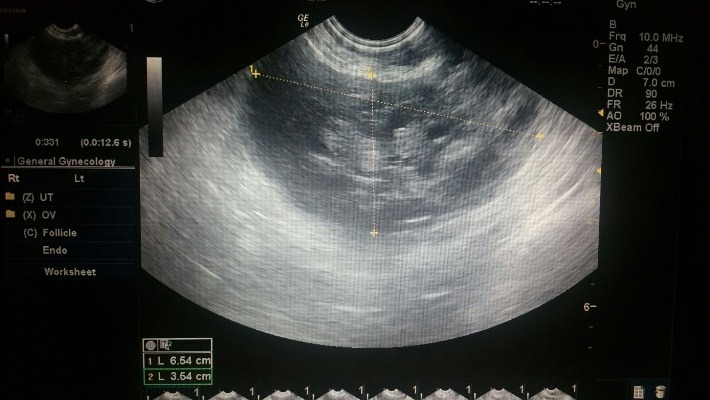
Ultrasound image of postpartum perirectal hematoma
